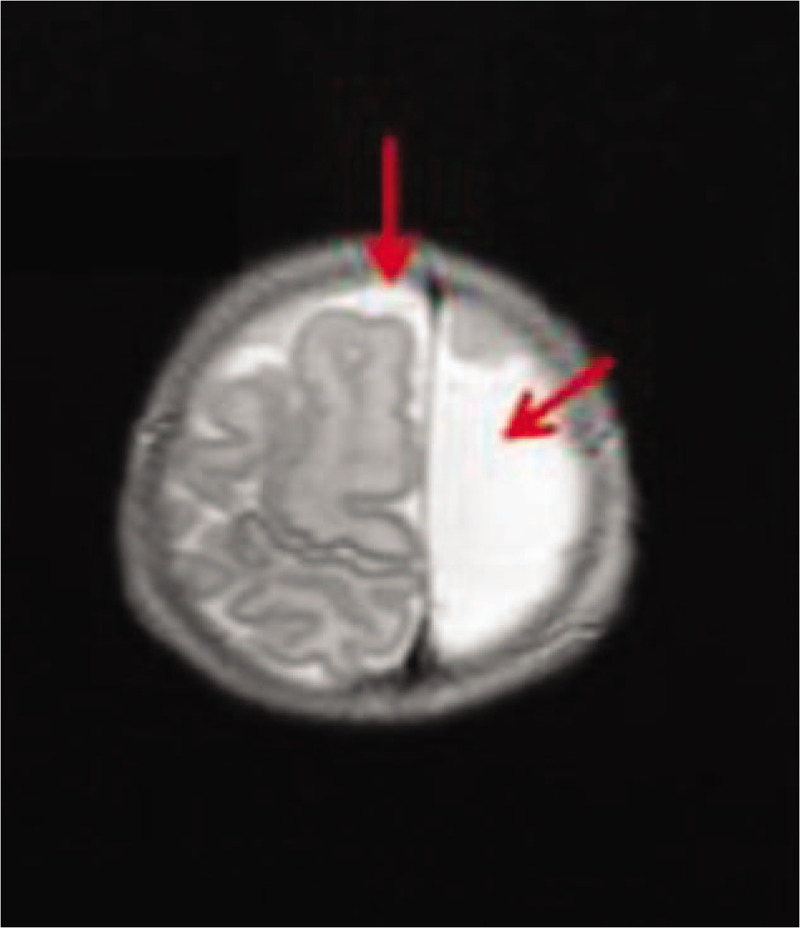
Brain magnetic resonance imaging. Asymmetry of cerebral hemispheres with hypoplasia of left cerebral hemisphere, dilatation of left ventricle (red flags) and porencephaly.
